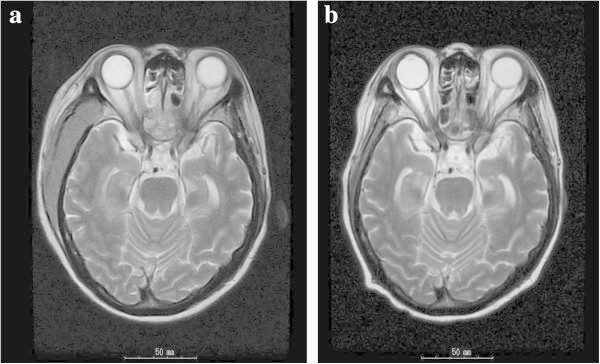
MRI images of the head. a The extra-medullary mass of right temporal region before dasatinib treatment. b Forty-six days after initial dasatinib treatment.
